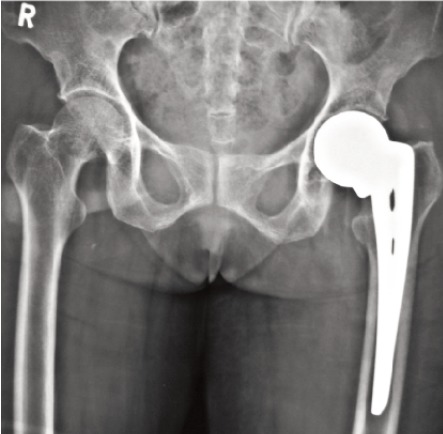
Post operative radiograph of unipolar prosthesis
